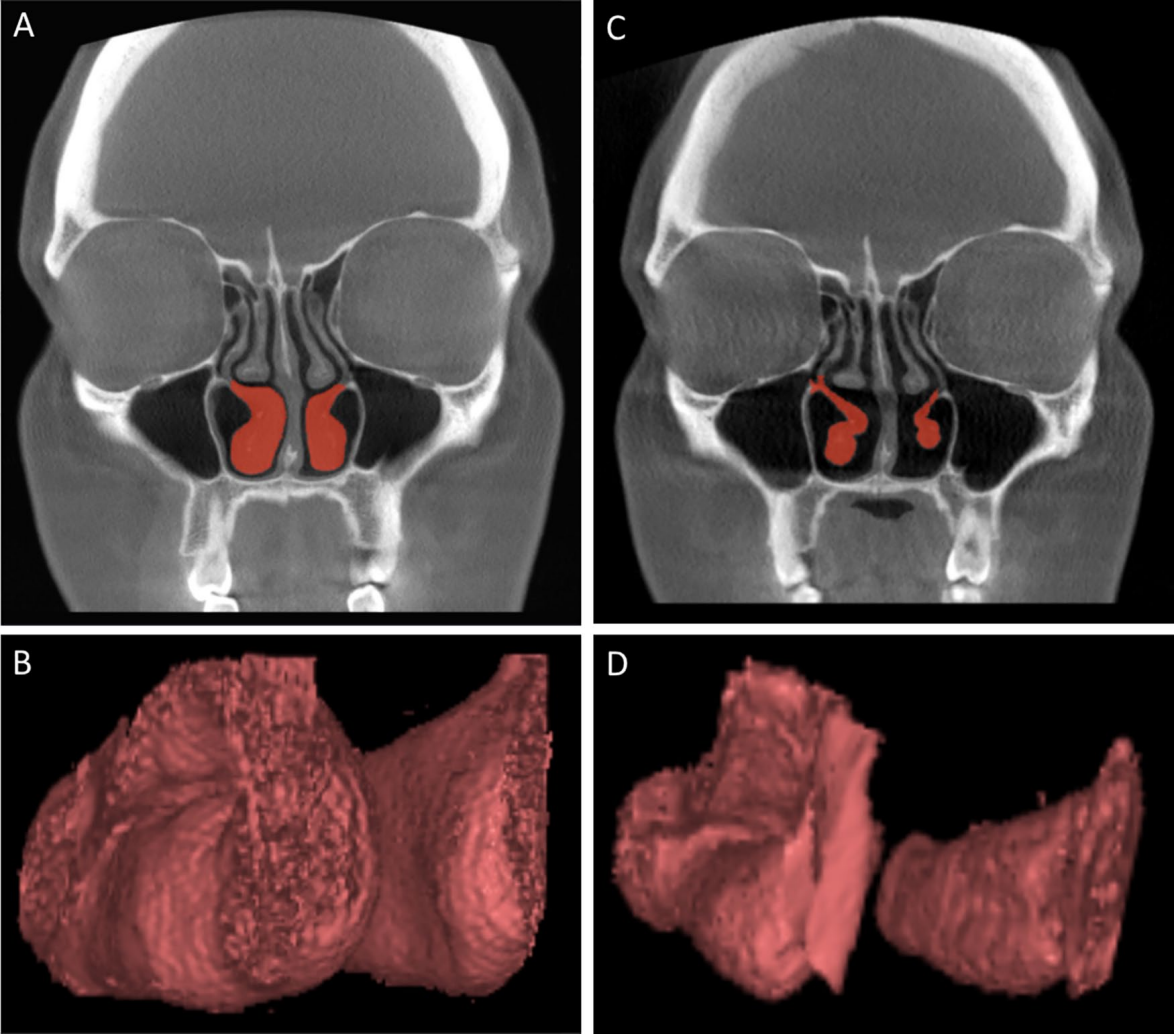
An example of the preoperative patient CBCT scans (A) with marked inferior
